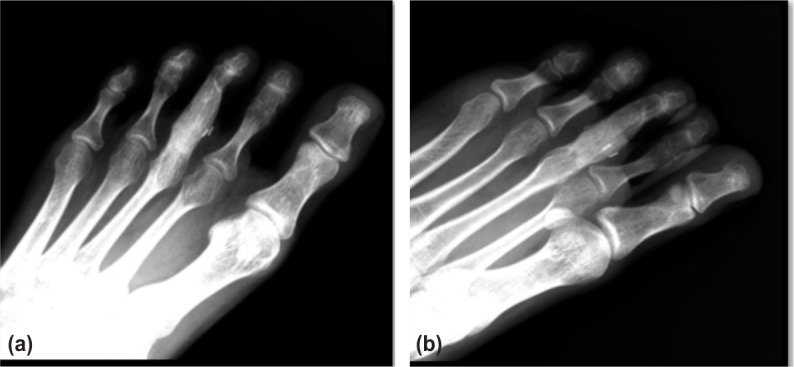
Left foot radiograph 8 months post surgery shows good healing process with no local recurrence.
